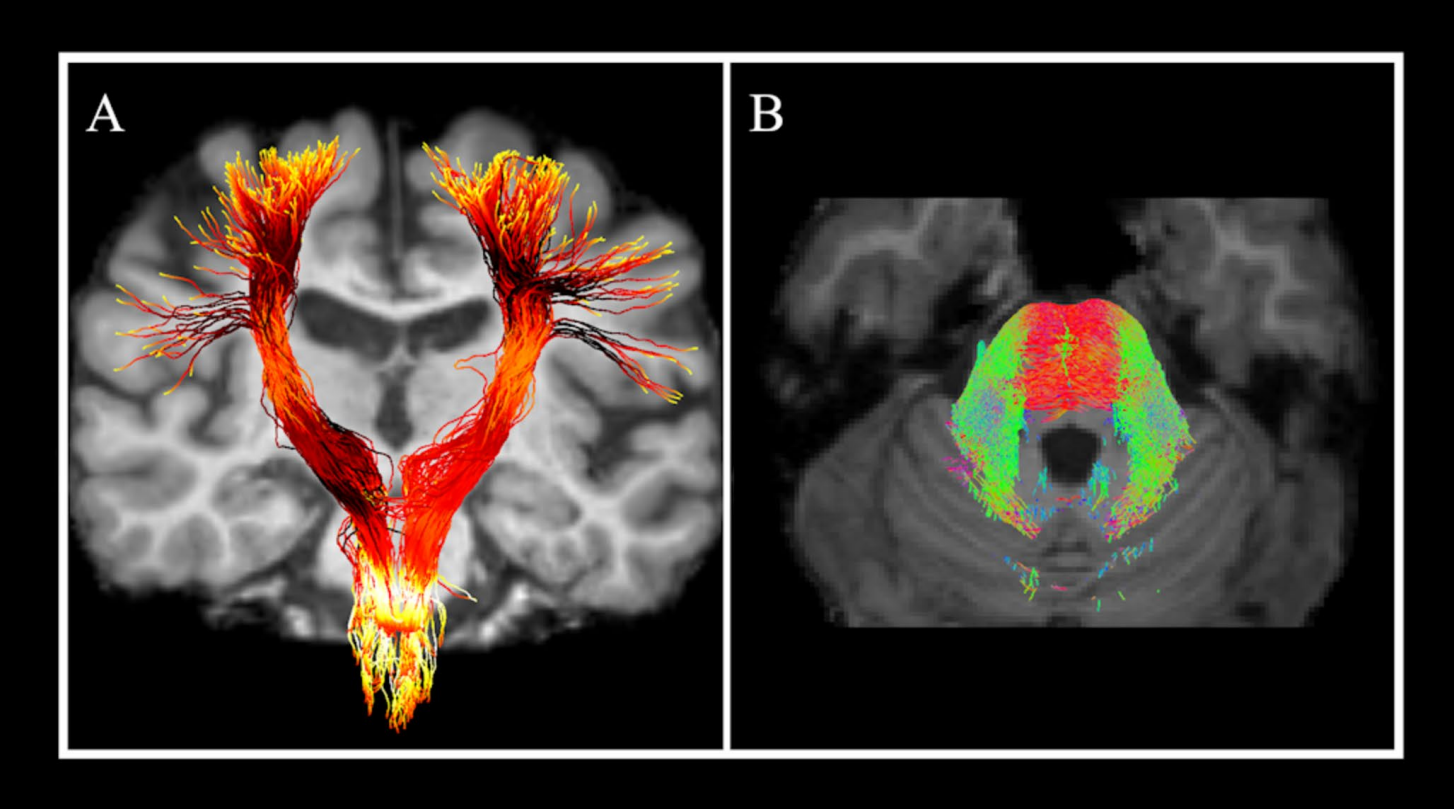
An example of advanced imaging findings in ARSACS. Tractography reconstruction from a diffusion MRI sequence using a probabilistic approach of the corticospinal tract (A) and of the bundles at the level of the pontine region (B), with the latter image showing an over-representation of the latero-laterally oriented fibres that fill nearly the whole pons (*red*), along with thickened middle cerebellar peduncles (*green*) in an ARSACS patient
